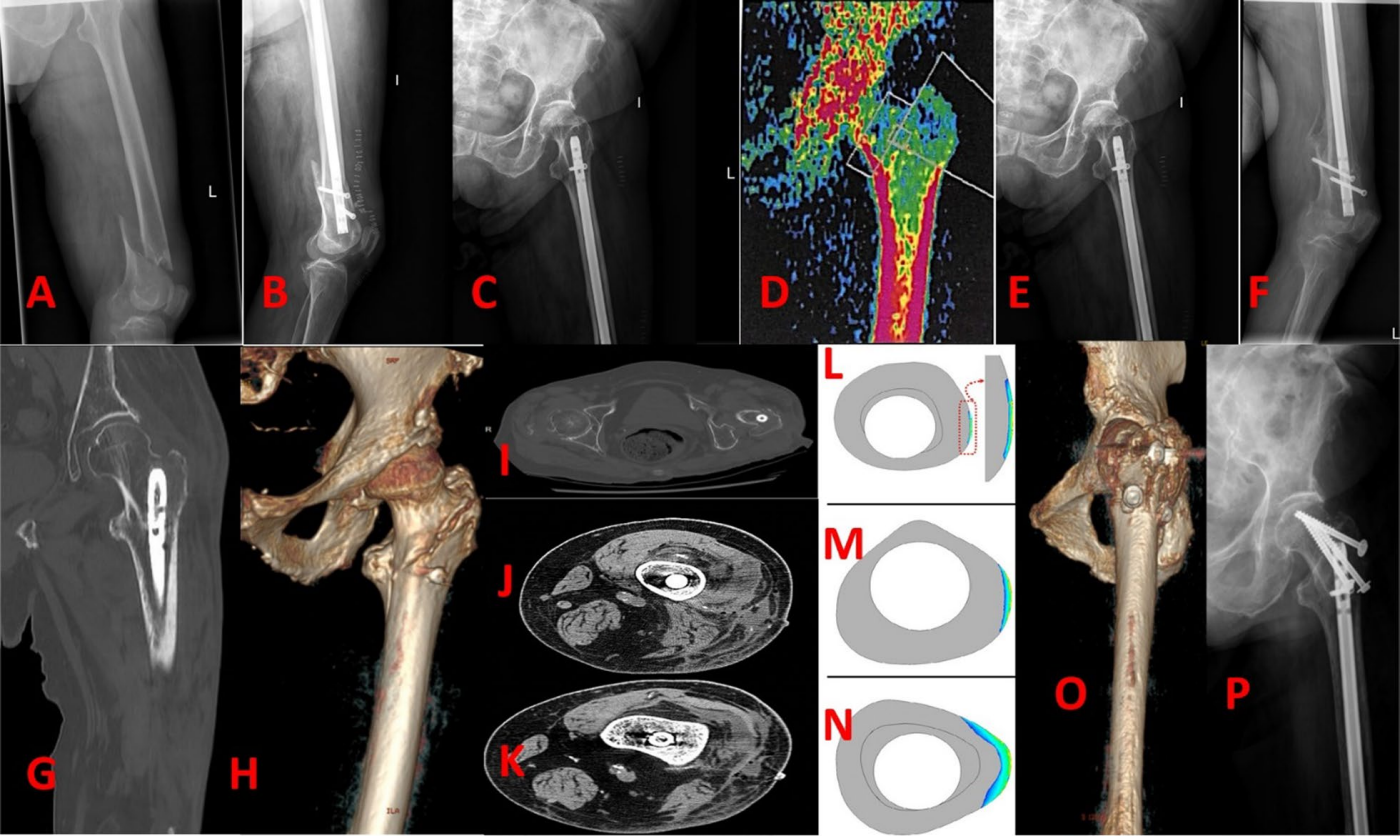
Forty-eight-year-old female patient sustaining a distal third femoral fracture (A). Surgical treatment of the same patient with blocked retrograde intra-medullary nail (B, C). DEXA scan 1 year after surgery showing osteoporosis at the level of the trochanters and femoral diaphysis (D). Femur X-ray 1 year after surgery shows femur demineralization of the proximal femoral third (E) and complete fracture union at the distal femoral third (F). Two months after the latest follow-up visit, the patient presented to the local hospital after a fall, complaining of ipsilateral hip pain. Femur X-ray and CT scan highlighted an undisplaced intertrochanteric fracture (G, H). CT scan cuts at the level of the greater trochanter (I), isthmus (J) and condyles (K) are compared with biomechanical studies belonging to Shih et al. [22] (L–N) and show shielding of the nail with stress applied to the intertrochanteric area. The intertrochanteric fracture was surgically managed with three cannulated screws. 6 months post-operation X-ray and CT scan showed fracture union (O, P)
